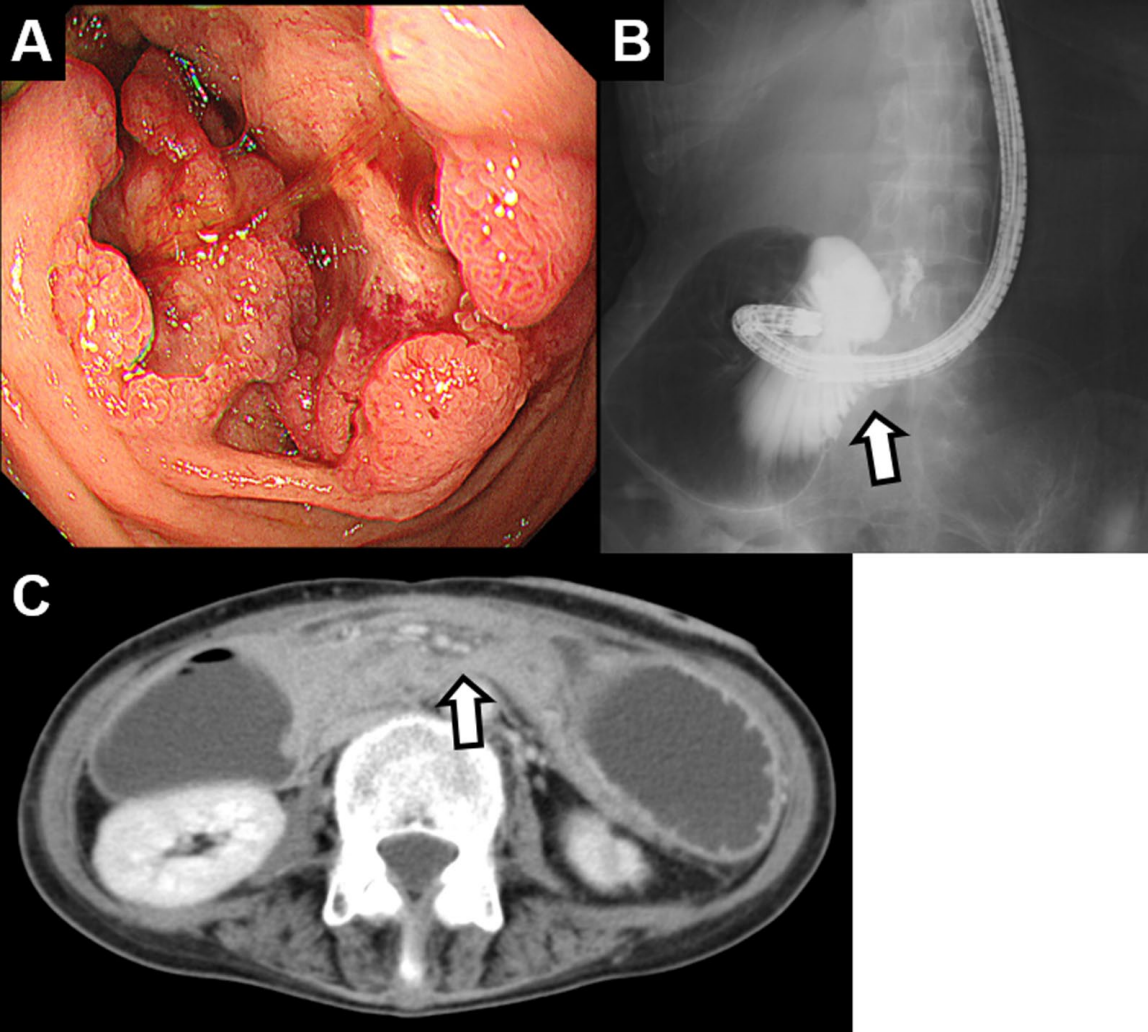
A Upper gastrointestinal endoscopy revealing a type 2 tumor in the third duodenal portion. B Upper gastrointestinal series revealing that contrast medium was unable to pass the third duodenal portion. C Computed tomography revealing the stricture in the third duodenal portion, but no mass could be identified. The stomach and duodenum are shown dilated
